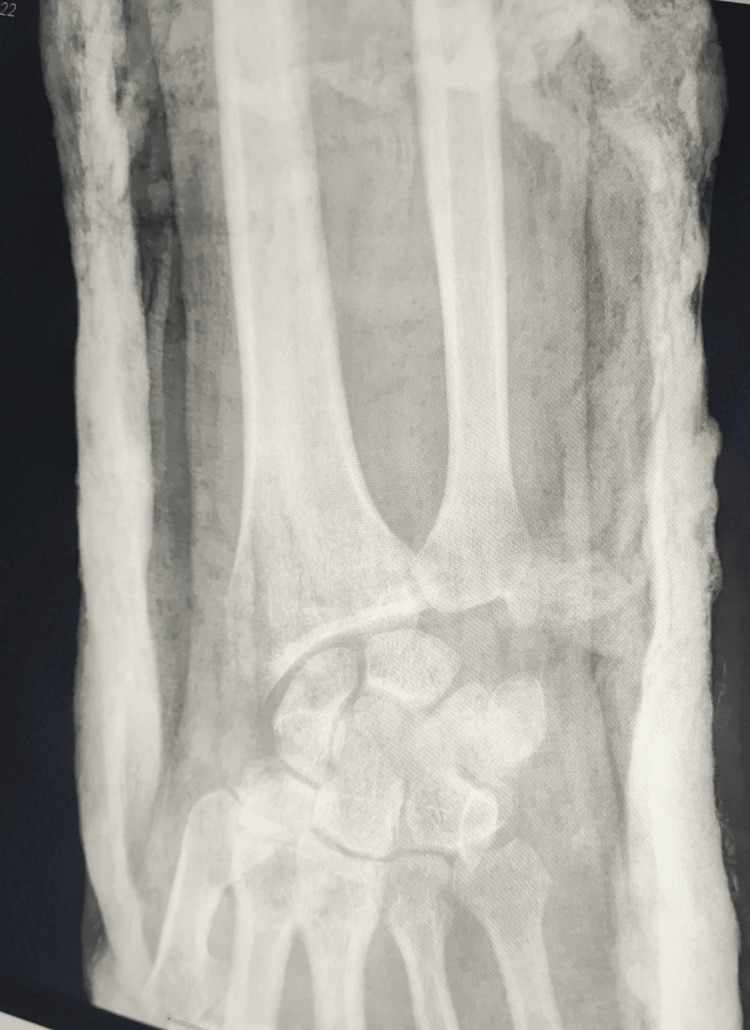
Post‑reduction anteroposterior X-ray Post‑reduction anteroposterior wrist radiograph demonstrating proper alignment of the nondisplaced ulnar styloid fracture.
